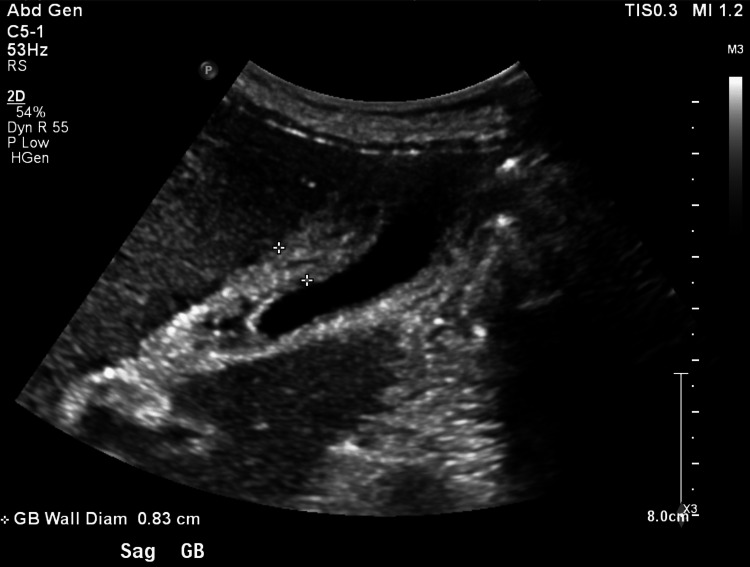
Abdominal ultrasound showing edematous gall bladder with some pericholecystic fluid.
